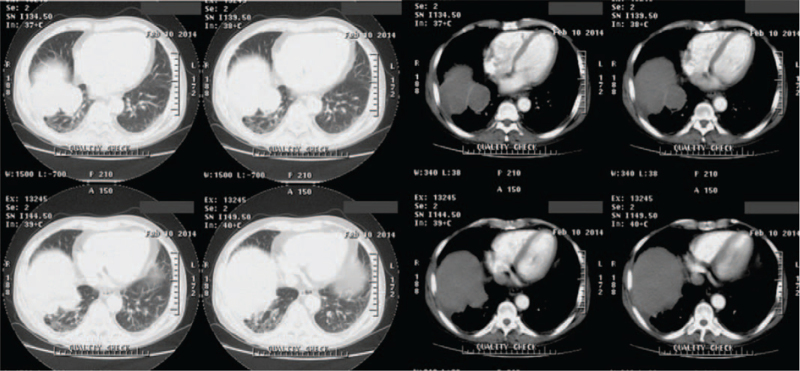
Two, large, well-defined masses occupying the inferior lobe of right lung, along with slight pleural thickening and little pleural effusion are showed by the enhanced chest CT. CT = computed tomography.
